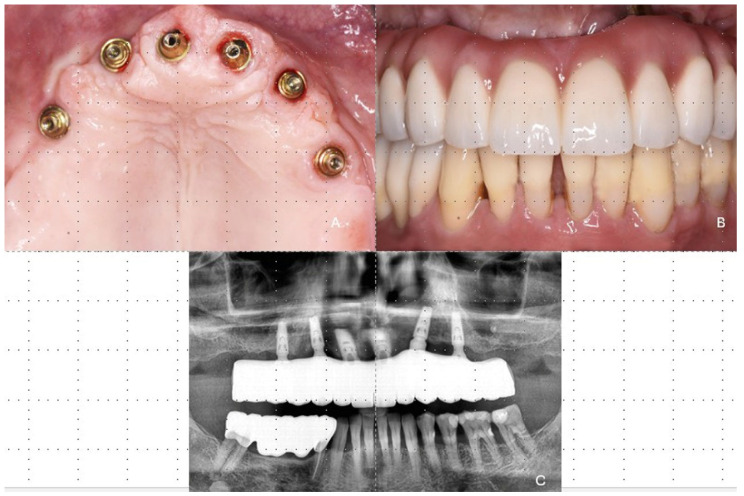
(A,B) Clinical situation at 1-year recall, (C) Radiographic examination at 1-year recall.
